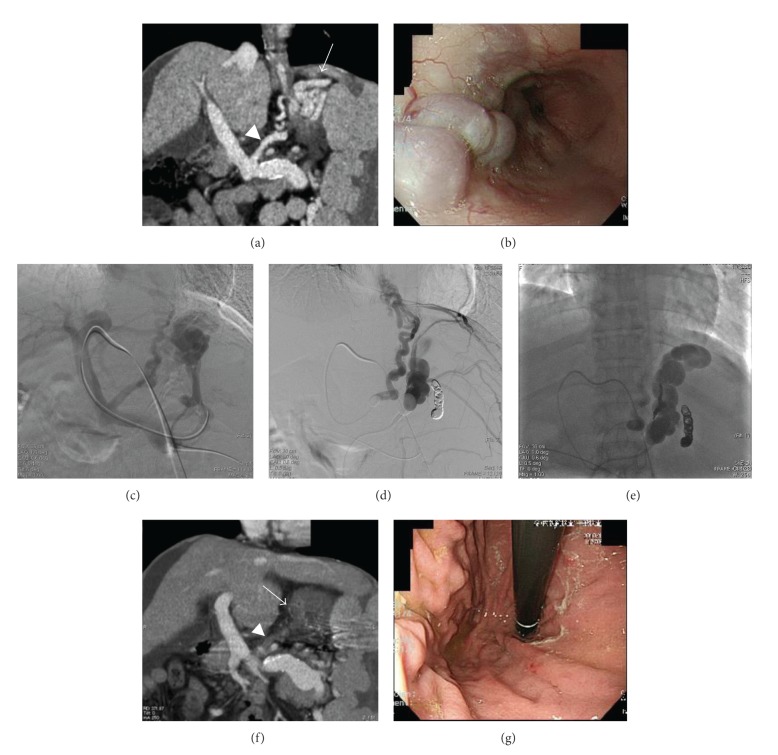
A 36-year-old woman with gastroesophageal varices. The patient visited our outpatient clinic because of general fatigue. Hematologic tests showed liver dysfunction and pancytopenia, and the gastroesophageal varices tended to worsen despite several sessions of endoscopic therapy. An enhanced computed tomography (CT) scan coronal image (a) showed dilated and tortuous veins at the gastric fundus (arrow), and large gastric varices supplied by the left gastric vein (arrowhead). Endoscopic examination of the stomach (b) showed significant large tumorous gastroesophageal varices (F3). Such varices pose a high risk of variceal bleeding and are an indication for embolotherapy. This patient underwent combined balloon-occluded retrograde transvenous obliteration (BRTO) and PTO therapy. Direct portography (c) showed gastroesophageal varices from the left gastric vein and posterior gastric vein. Balloon-occluded left gastric venography and left adrenal venography after embolization of the posterior gastric vein with platinum coils showed the left inferior phrenic vein and intercostal vein as collateral vessels (d). Fluoroscopic image obtained at 4 hours after embolotherapy shows the varices filled with iopamidol (e). An enhanced coronal CT scan obtained 14 days after embolotherapy showing the gastric varices and the left gastric vein as a low-density area ((f), arrow and arrowhead), suggesting complete obliteration. Endoscopic examination conducted 6 months after the embolotherapy showed eradication of the gastroesophageal varices (g).
